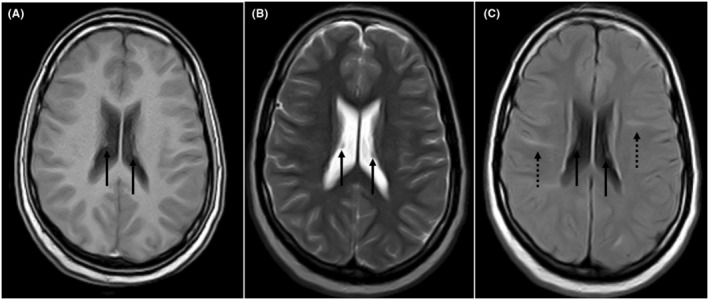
Axial T1W (A), T2W (B), and FLAIR (C) images showing small subependymal nodules (black arrows) and FLAIR hyperintense radial bands (dotted arrow, C).
